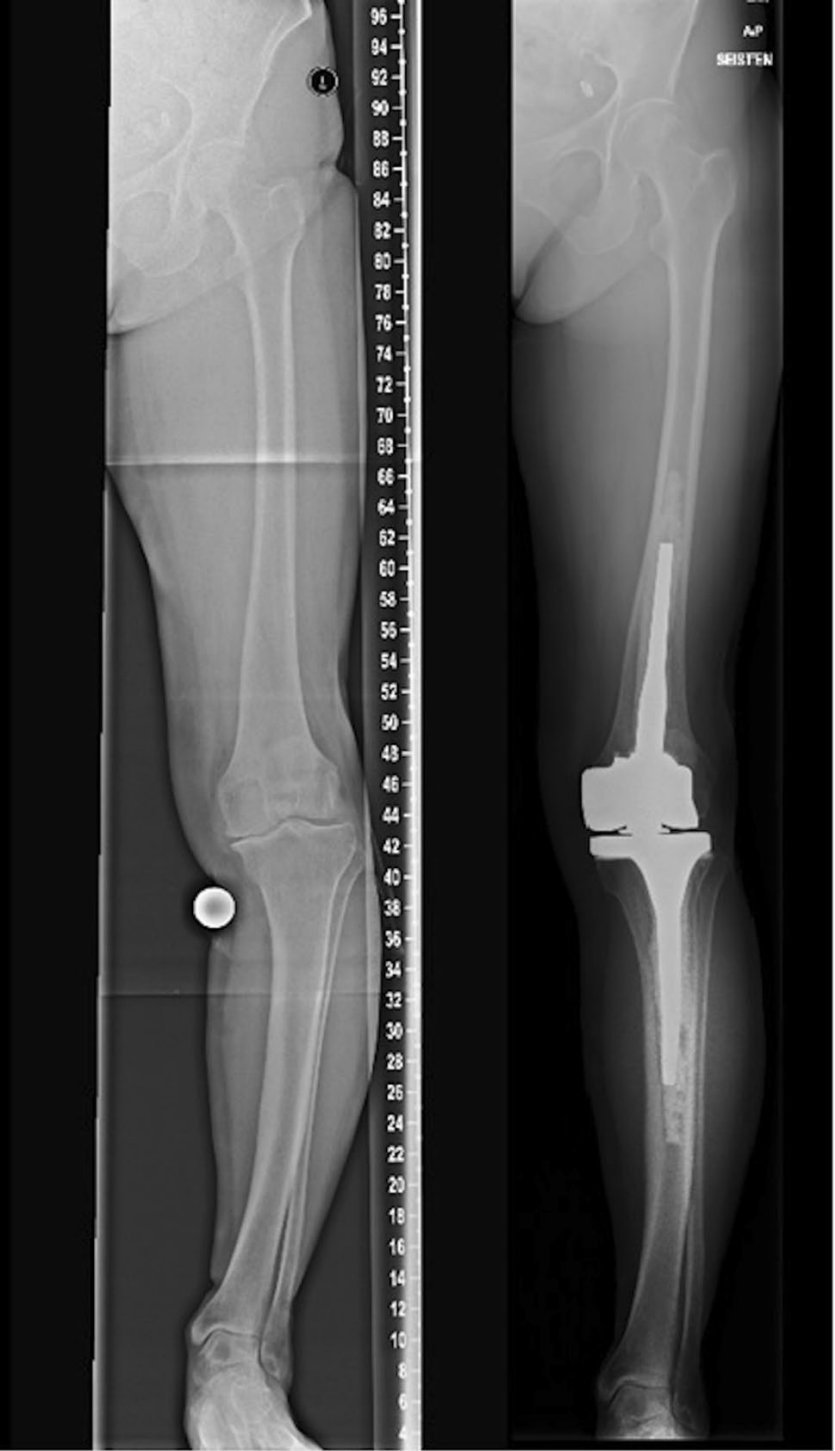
Patient with unknown skeletal disorder. Pre- and post-operative long leg weight-bearing radiographs of a patient with an unknown skeletal disorder that led to a curved tibia. The surgeon considered other options, such as patient-specific instrumentations, but ended up deciding on a CMH. MA was corrected to normal, and OKS was 45
